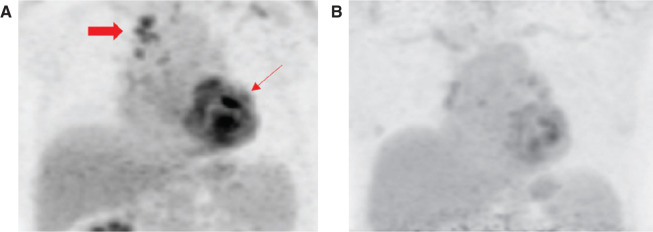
Cardiac ^18^F-FDG-PET scan showing myocardial 18F-FDG uptake. A: Extensive abnormal heterogeneous ^18^F-FDG myocardial uptake (thin arrow) and uptake of mediastinal lymph nodes (wide arrow). B: Overall decreased ^18^F-FDG uptake 10 months after the initial procedure following immunosuppressive therapy.
